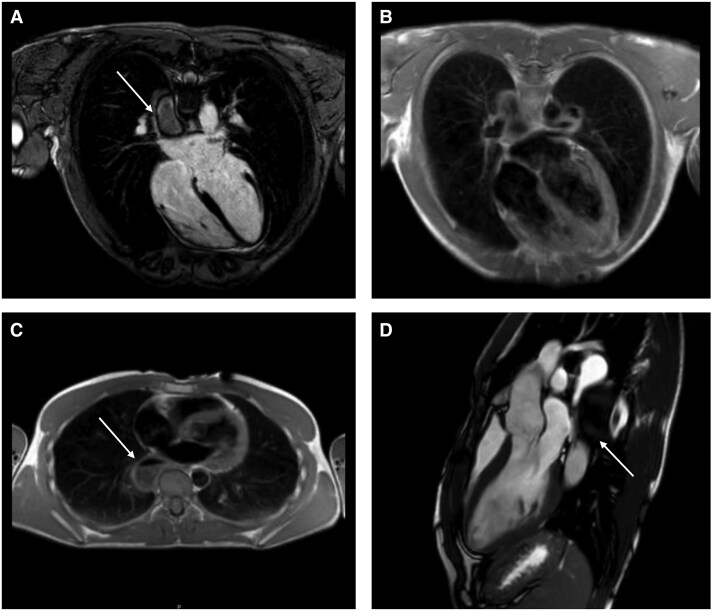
Magnetic resonance findings. (*A*) Cine steady-state free precession cardiac MR image (axial view) showing an apparent mass along the posterior wall of the left atrium (arrow). (*B*) T1-weighted black-blood axial image showing a structure (arrow) located posteriorly to the left atrium, with signal intensity consistent with the oesophageal lumen. (*C*) T1-weighted black-blood axial cardiac MR image demonstrating a tubular structure (arrow) posterior to the left atrium, consistent with the oesophageal lumen rather than an intracavitary mass. (*D*) Sagittal cine steady-state free precession image showing the left atrium and the adjacent bright oesophageal lumen posteriorly
